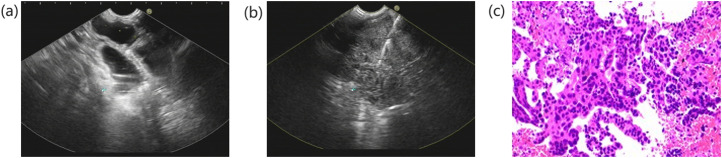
(a) A pelvic hypoechoic mass, approximately 9 cm in diameter, was discovered by ultrasound endoscopy. (b) A fine-needle aspiration biopsy was conducted on the pelvic mass under the guidance of endoscopic ultrasonography. (c) The solid pelvic mass’s pathologic results revealed cancer.
